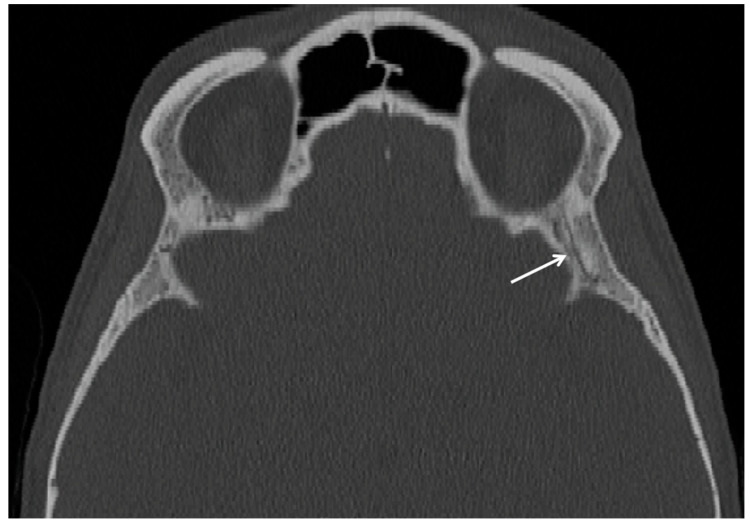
Tranverse CT scan of an individual with the left foramen meningo-orbitale (white arrow).
